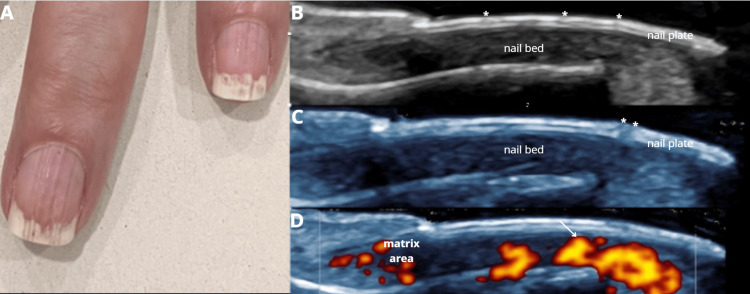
Chronic use of acrylic nails mimicking psoriasis: pseudopsoriatic ultrasound pattern. (A) Clinical image of a 59-year-old female patient with uninterrupted use of acrylic nails for three years. Following removal, clinical findings, including roller-coaster transverse onycholysis with a proximal erythematous border and onychorrhexis, raised suspicion of psoriasis, which required exclusion. (B) and (C) Longitudinal grayscale ultrasound images showing preserved trilaminar architecture of the nail plates. However, hypoechoic vertical and oblique bands (indicated by asterisks) traverse the entire lamina, consistent with chronic microtrauma. No ultrasound signs of psoriasis are present, such as hyperechoic foci with posterior acoustic shadowing or thickening of the nail bed. (D) Longitudinal power Doppler image showing hypervascularization (white arrow) beneath the ventral plate, reflecting chronic inflammation. These findings are consistent with pseudopsoriatic changes secondary to chronic acrylic nail use.
